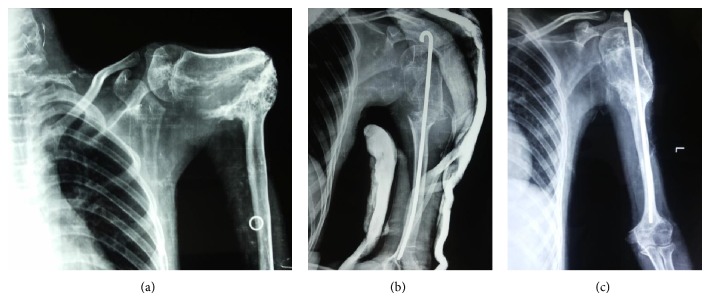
(a) Preoperative X-ray showing the lesion and the degree of deformity which at first appeared to the treating team to be an enchondroma. (b) Immediate postoperative X-rays in U slab. (c) X-rays at final follow-up showing union.
